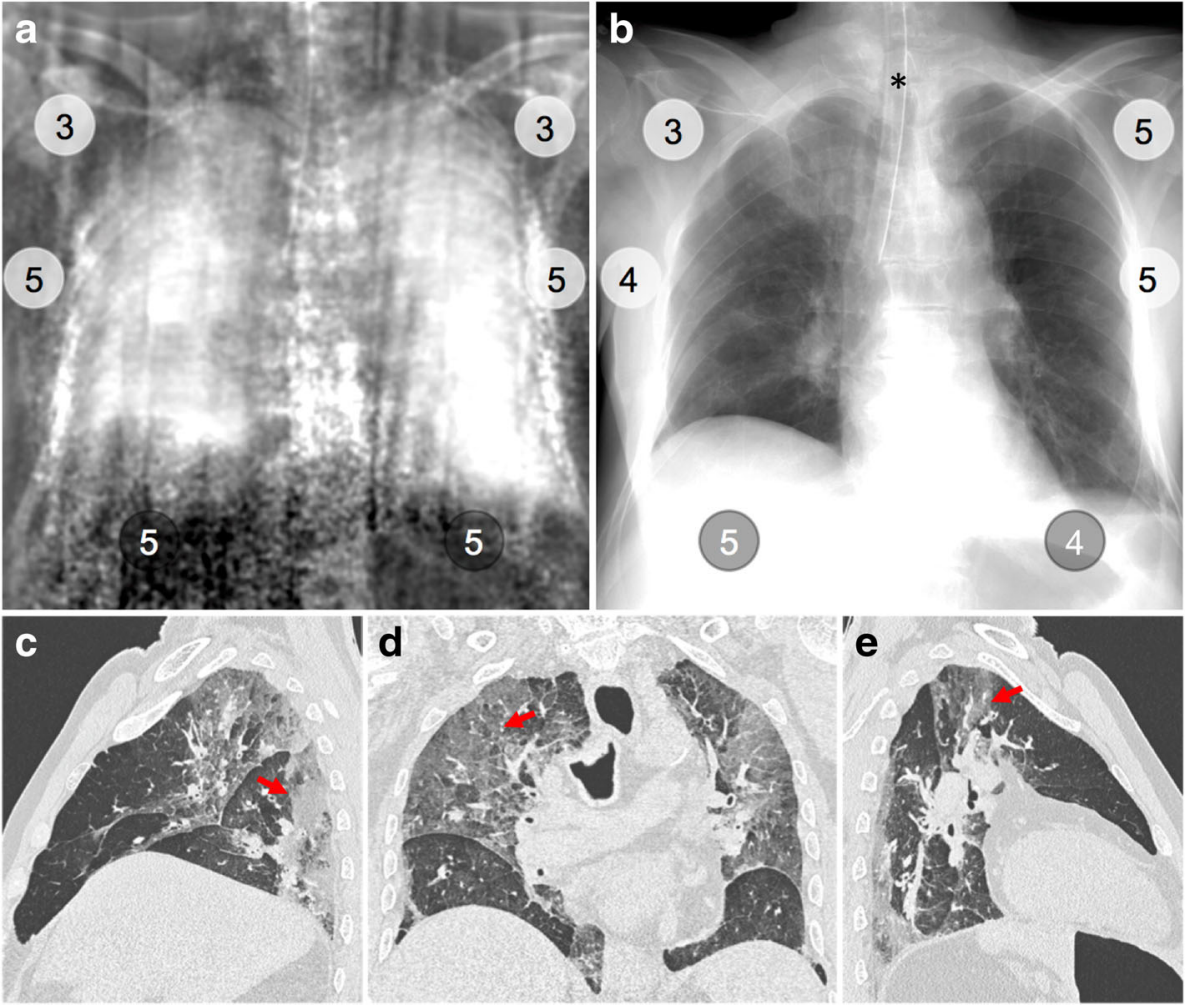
Dark-field chest radiograph, conventional x-ray, and CT of human body 3. Median visual grading (encircled numbers) of dark-field chest radiograph (a) by three independent readers shows highest dark-field signal in the middle and lower zones of the lung. Median visual grading of transmission in conventional x-ray (b) shows reduced transmission in the right lung upper zone and more pronounced in the left lung lower zone. Sagittal (c, e) and coronal (d) CT images show interstitial and alveolar infiltrates (red arrows) in dependent parts of right and left upper and lower lobes and right middle lobe. Asterisk marks the location of the endotracheal tube
